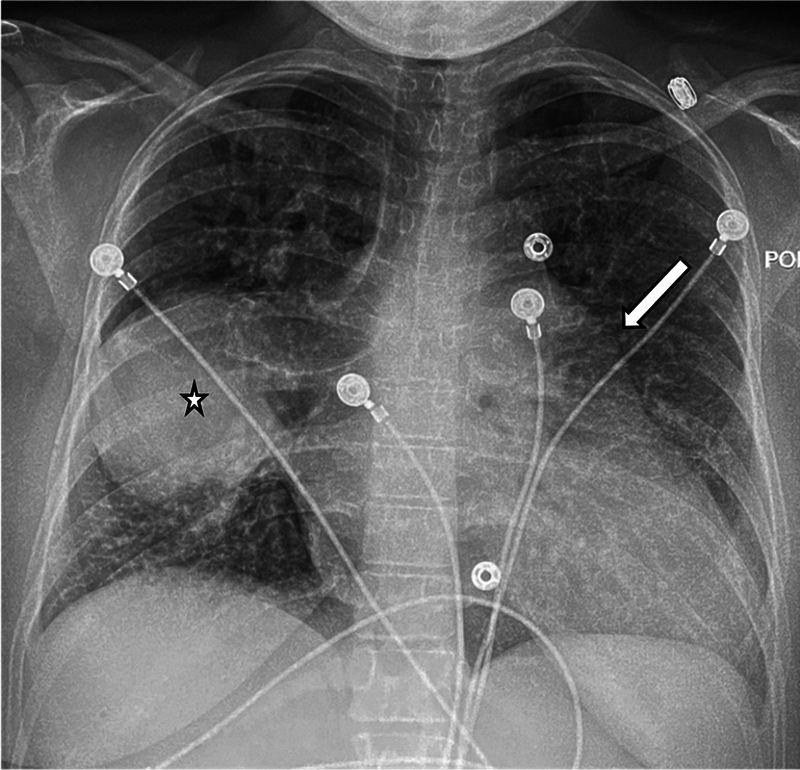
Frontal chest radiograph shows cardiomegaly, enlarged pulmonary artery (arrow), and a mass-like opacity in the right hemithorax (star) with well-demarcated lateral margin, broad-based toward the mediastinum with hilum convergence sign.
